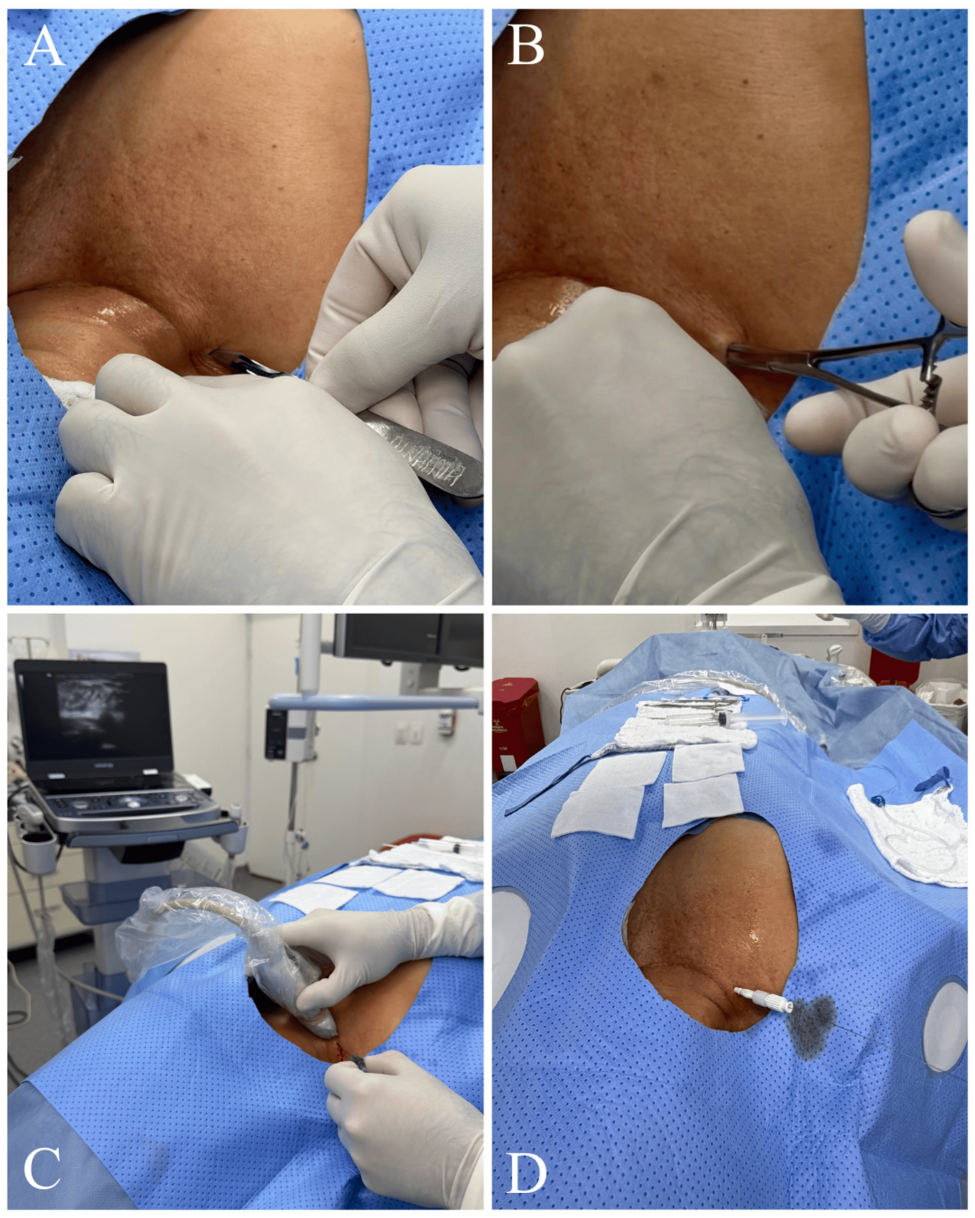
Venous access technique under ultrasound guidance (A) A 3-mm skin incision was made to facilitate access through the subcutaneous tissue. (B) Blunt dissection of the soft tissues was performed using mosquito forceps. (C, D) Under color Doppler ultrasound guidance at the supraclavicular level, venous access was obtained using a micropuncture set.
